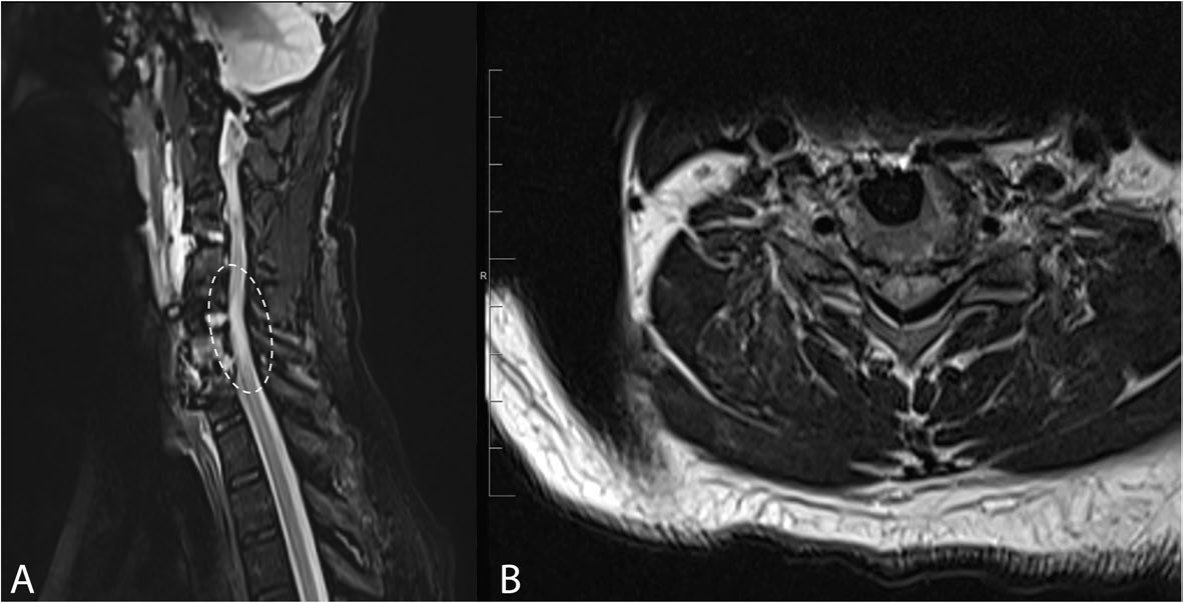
Post-operative sagittal STIR (A) and axial T2-weighted (B) MRI image showing cord swelling and abnormal high signal intensity at the levels C5 to C7 (encircled), representing changes of white cord syndrome
